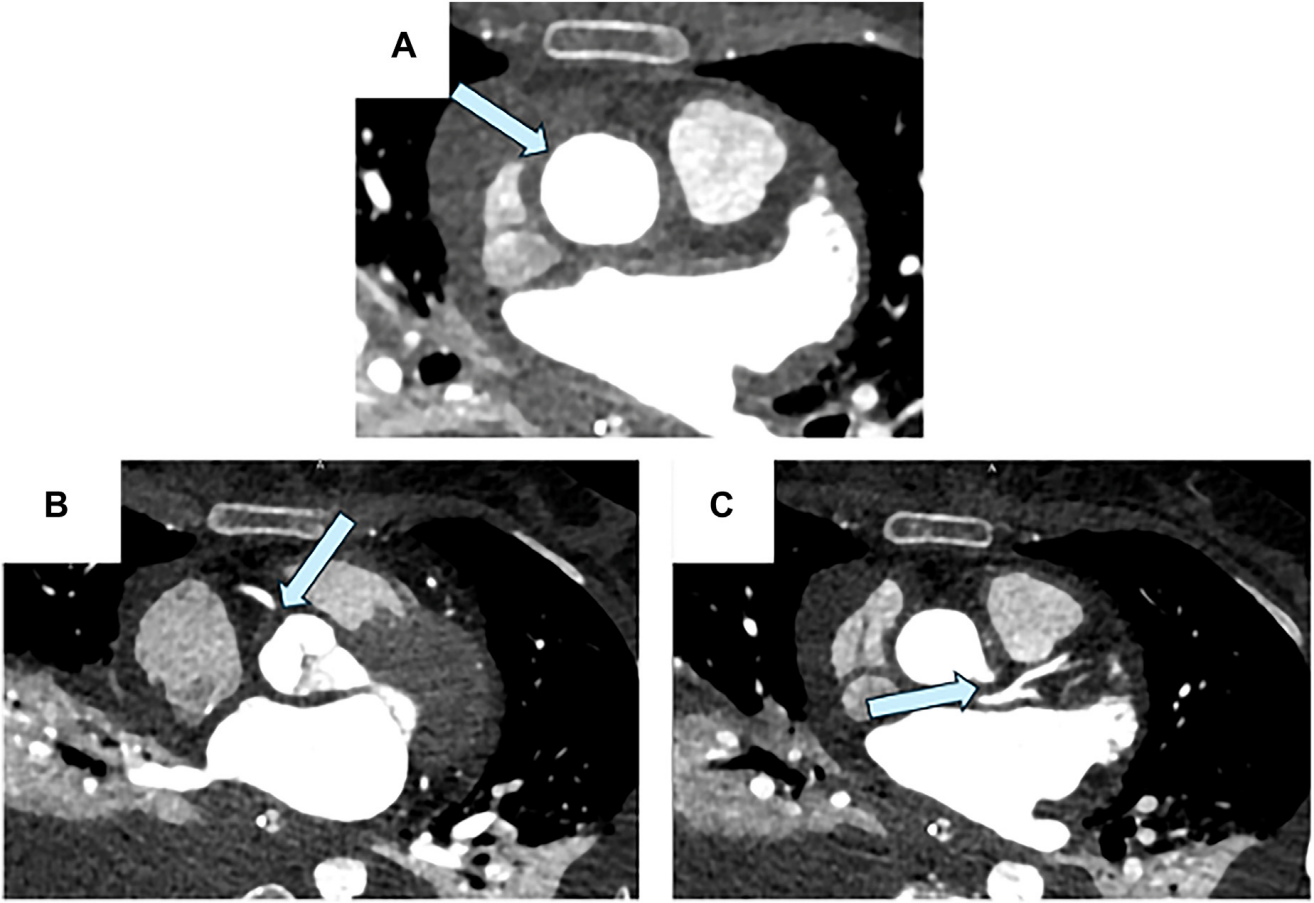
Preoperative retrogated computed tomography reveals coronary ostial stenosis secondary to syphilitic aortitis. Arrows show (A) circumferential wall thickening of the ascending aorta, (B) nearly complete occlusion of the right coronary artery, and (C) nearly complete occlusion of the left coronary artery.
